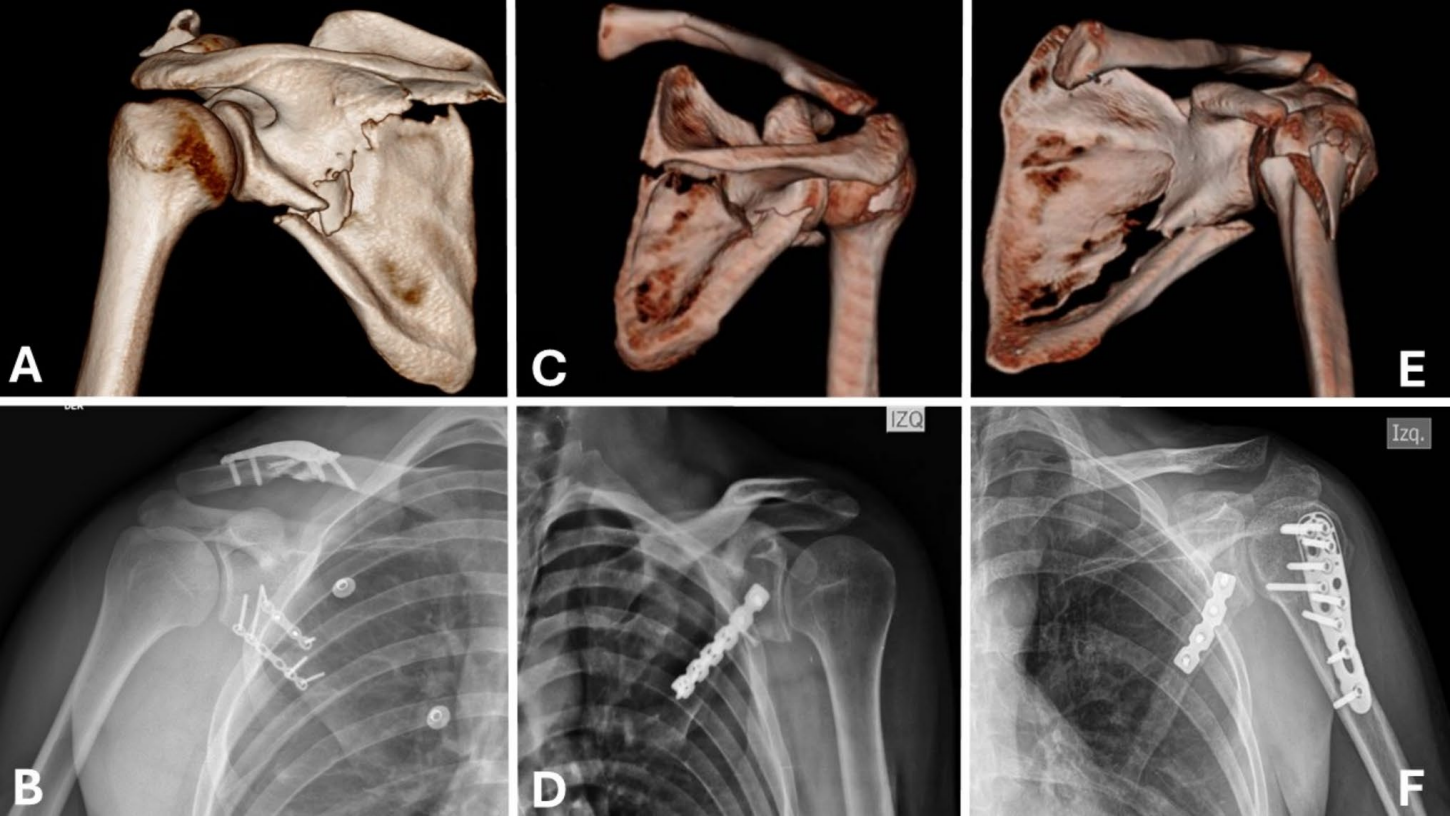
Radiological images showing the pre-and postoperative period of some patients. A Three-dimensional (3D) reconstruction CT scan showing a 14-B1 scapula fracture with a midshaft clavicle fracture in the right shoulder. B AP view of the shoulder 24 h postoperatively of Fig. 3A. C CT scan 3D reconstruction demonstrating a 14-B1 fracture of the left shoulder. D AP view of the shoulder 24 h postoperatively of Fig. 3C. E CT scan 3D reconstruction demonstrating a 14-B1 fracture with a proximal humerus fracture in the left shoulder. F AP view of the shoulder 24 h postoperatively of Fig. 3E
